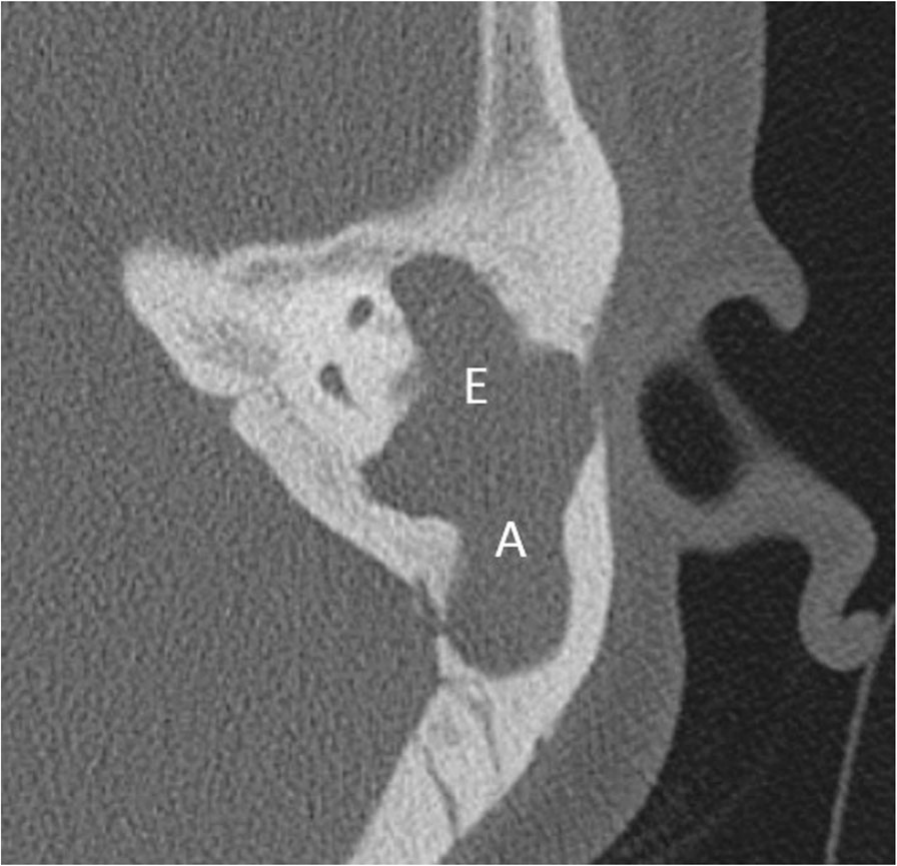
Axial CT reveals widening of aditus (denoted by A) and formation of common cavity between epitympanum (denoted by E) and aditus with soft tissue within
